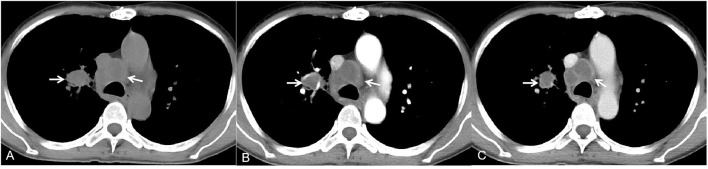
A 59-year-old man with rheumatic heart disease and recurrent cough, sputum and malaise for 3 months. The mediastinal window of the axial non-contrast-enhanced CT image showed multiple enlarged lymph nodes in the right hilus pulmonis and mediastinum [(A), white arrow]. Contrast-enhanced CT images showed lymph nodes with rim low enhancement [(B,C), white arrow].
